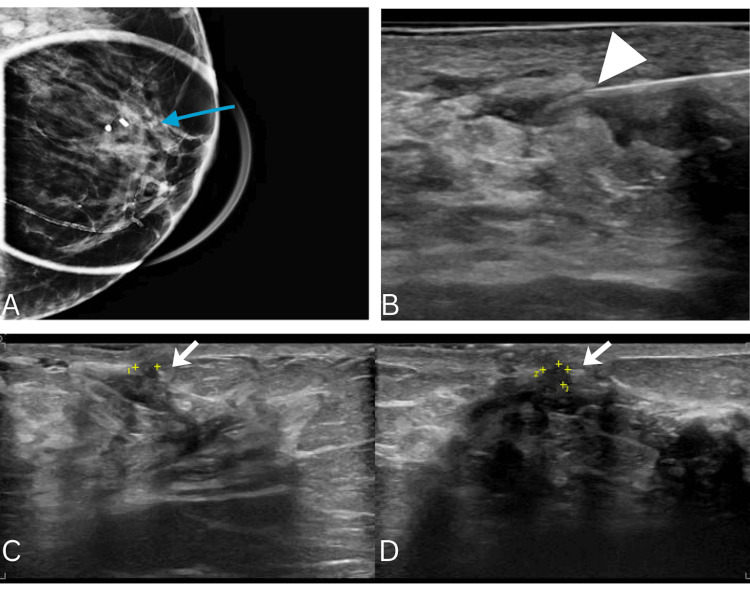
Diagnostic imaging of the left breast. (A) Left breast mammogram showing a suspicious palpable mass (blue arrow). (B) Ultrasound-guided biopsy of the mass (white arrowhead). (C-D) Ultrasound of superficial hypoechoic mass with ill-defined margins, 0.3 x 0.3 x 0.3 cm (white arrows).
